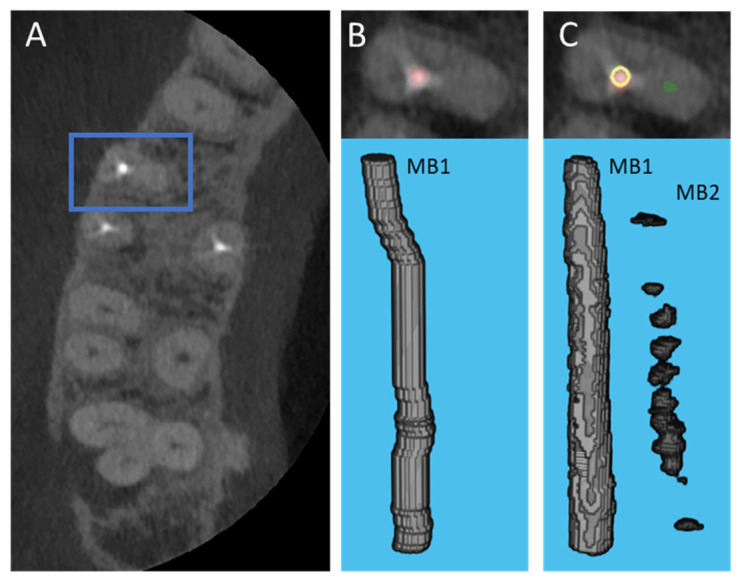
Case where U-Net performed better than the manual segmentation; an unobturated MB2 canal was present, yet the resident had difficulty segmenting the canal. (A) CBCT axial cross section view showing the location of the root, blue box indicates the root; (B) Manually generated segmentation visualized in the CBCT axial cross section (top) and the corresponding 3D view (bottom), the red color depicts the superimposed obturated MB1 canal; (C) U-Net generated segmentation visualized in the CBCT axial cross section (top) and the corresponding 3D view (bottom), the U-Net generated canal segmentation is red for the obturated MB1 canal and green for the unobturated MB2 canal, the yellow ring represents the location of manual segmentation relative to U-Net generated segmentation.
